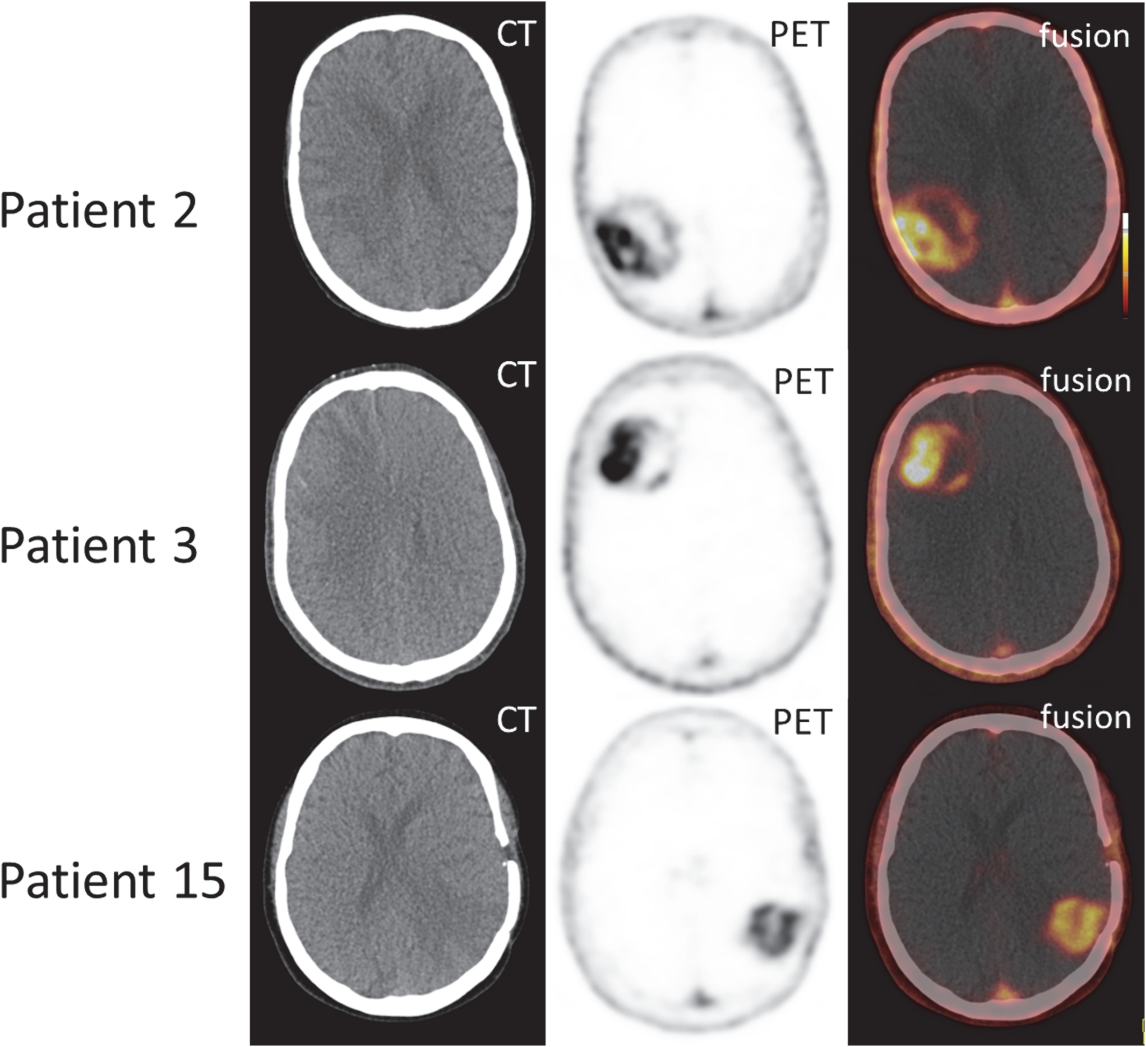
Tracer distribution of ^68^Ga-DOTATATE in GBM. Three examples of increased ^68^Ga-DOTATATE uptake in Glioblastoma multiforme. Markedly, partly inhomogeneous tracer accumulation can be depicted in each patient. Shown are axial views of CT, SSTR-PET as well as fused PET/CT images.
